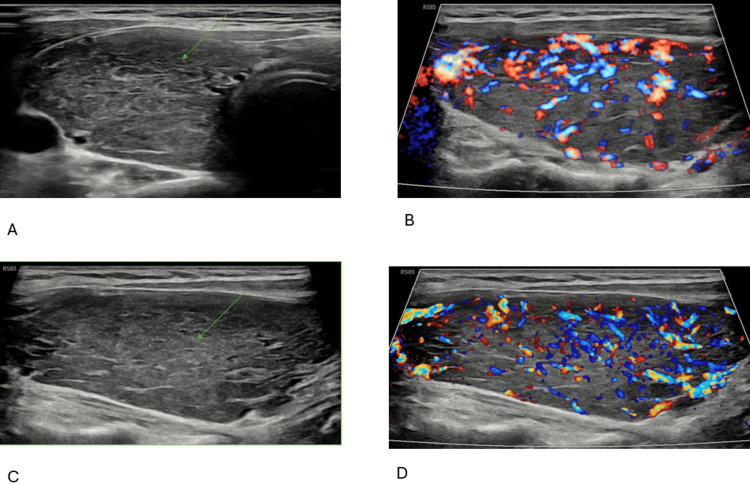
Thyroid ultrasound showed an enlarged, heterogeneous gland with florid vascularity A: plain ultrasound showing diffuse enlargement of the right thyroid lobe (arrow); B: color Doppler ultrasound showing increased vascularity of the right thyroid lobe; C: plain ultrasound showing diffuse enlargement of the left thyroid lobe (arrow); D: color Doppler ultrasound showing increased vascularity of the left thyroid lobe.
